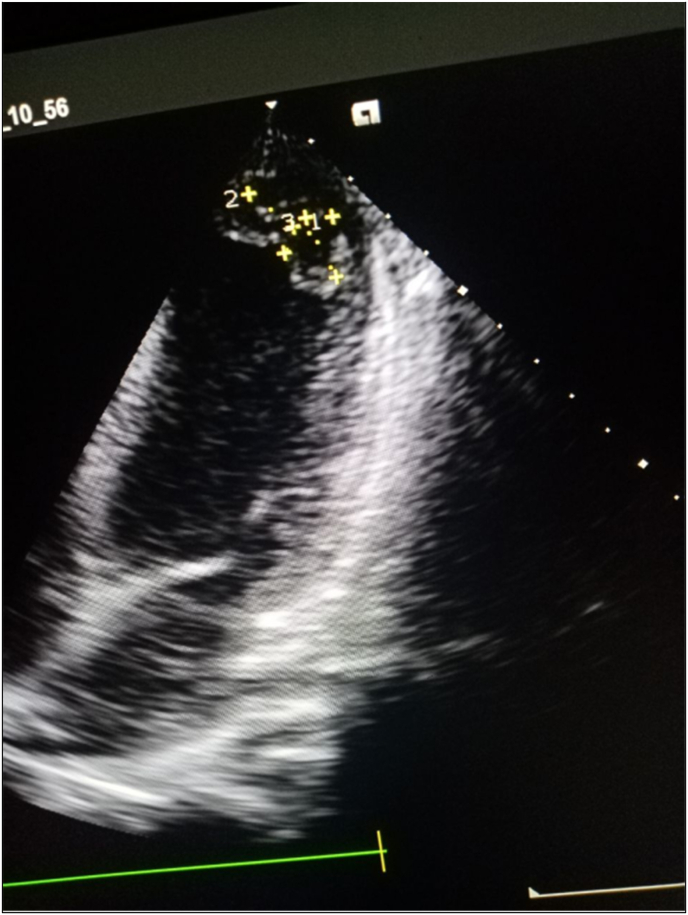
Echocardiography showed anterior, septal, apical and anterolateral wall hypokinesia.
